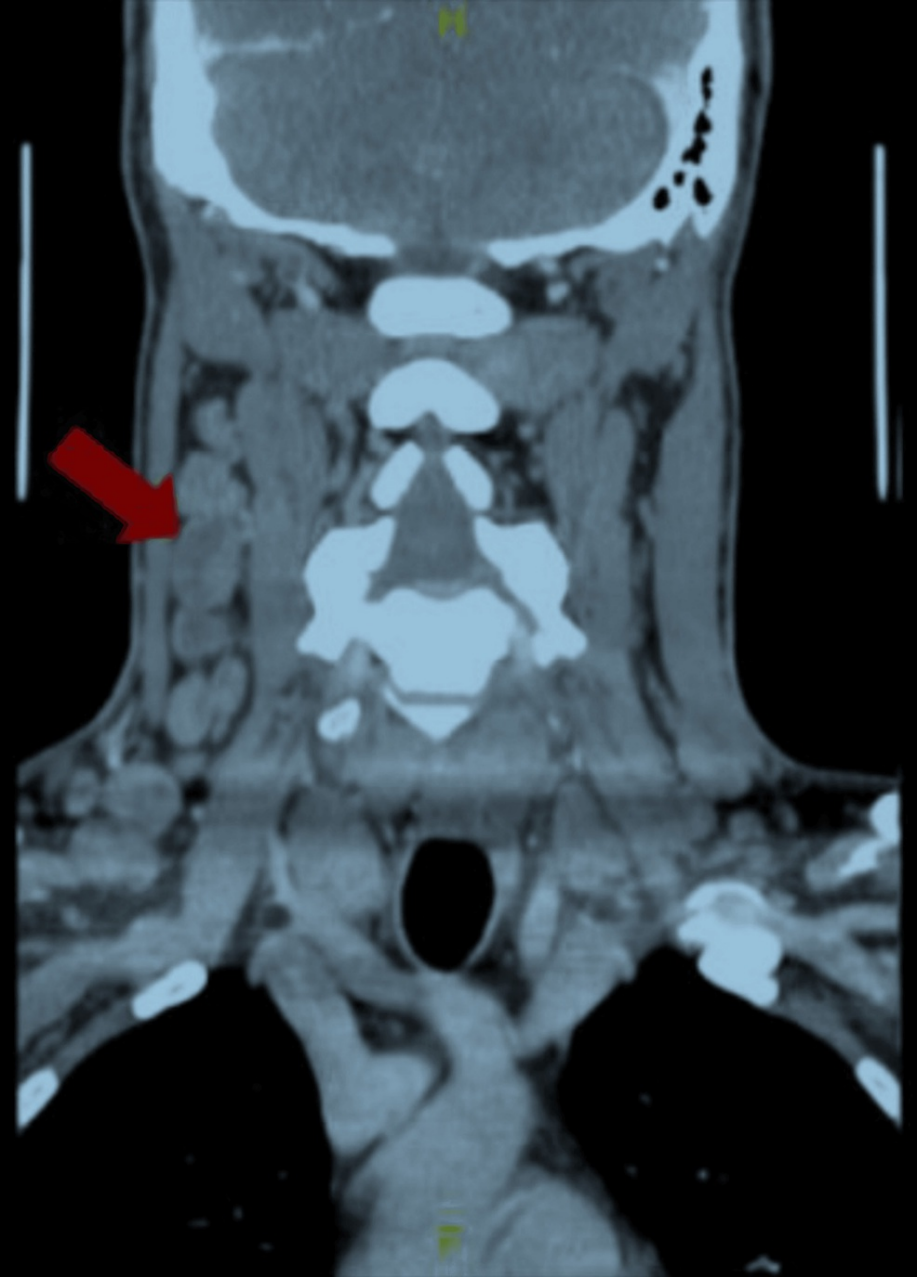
CT of the neck. This is a frontal plan of the patient’s CT of the neck showing multiple cervical adenopathy most pronounced on the right side of the neck (red arrows).
